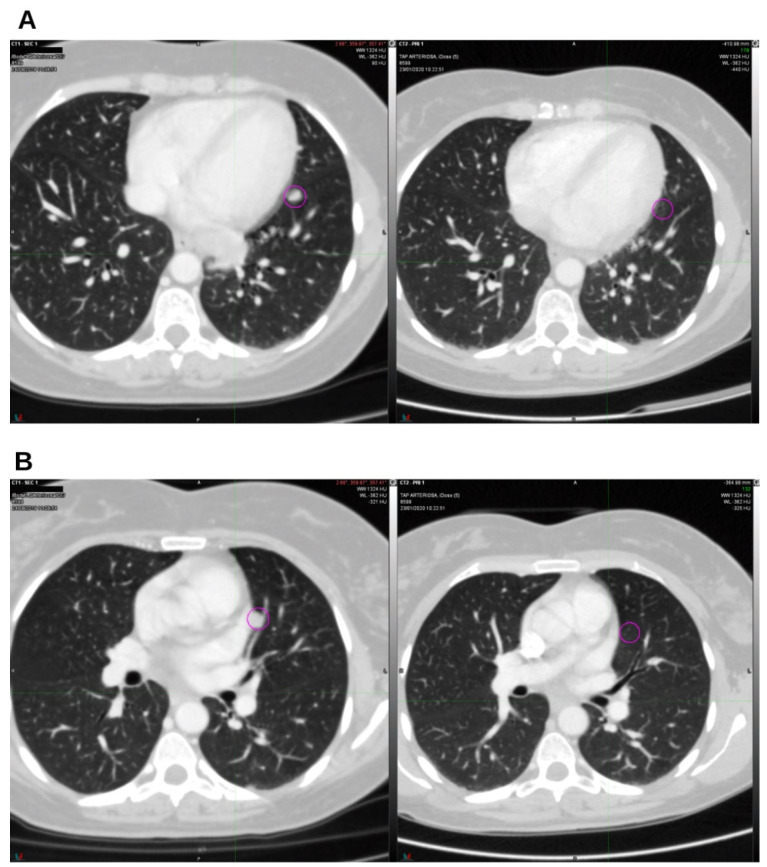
On the left, CT from August 2019; on the right, CT from January 2020. The lingular (A) and parascissural (B) nodules were in complete regression. Nodes in the circles.
